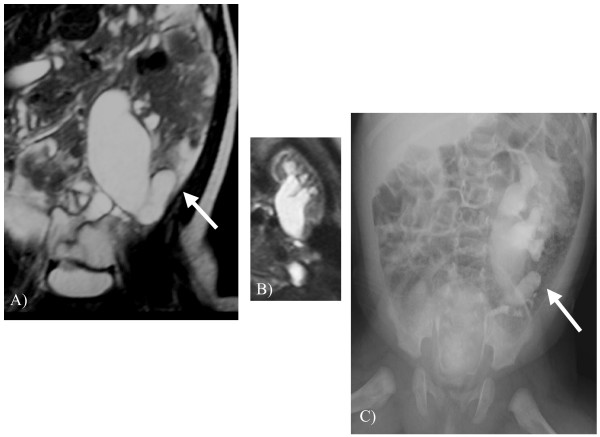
Magnetic Resonance Imaging (MRI) coronal T2-weighted images (Panel A), coronal MR urography (Panel B), and intrasurgical retrograde ureteropyelogram (Panel C). On coronal T2-weighted images and coronal MRI urography, can be depicted an obstruction of the middle third of the ureter, with upstream hydro-urethronephrosis, that was subsequently confirmed by intrasurgical retrograde ureteropyelogram (Panel C).
